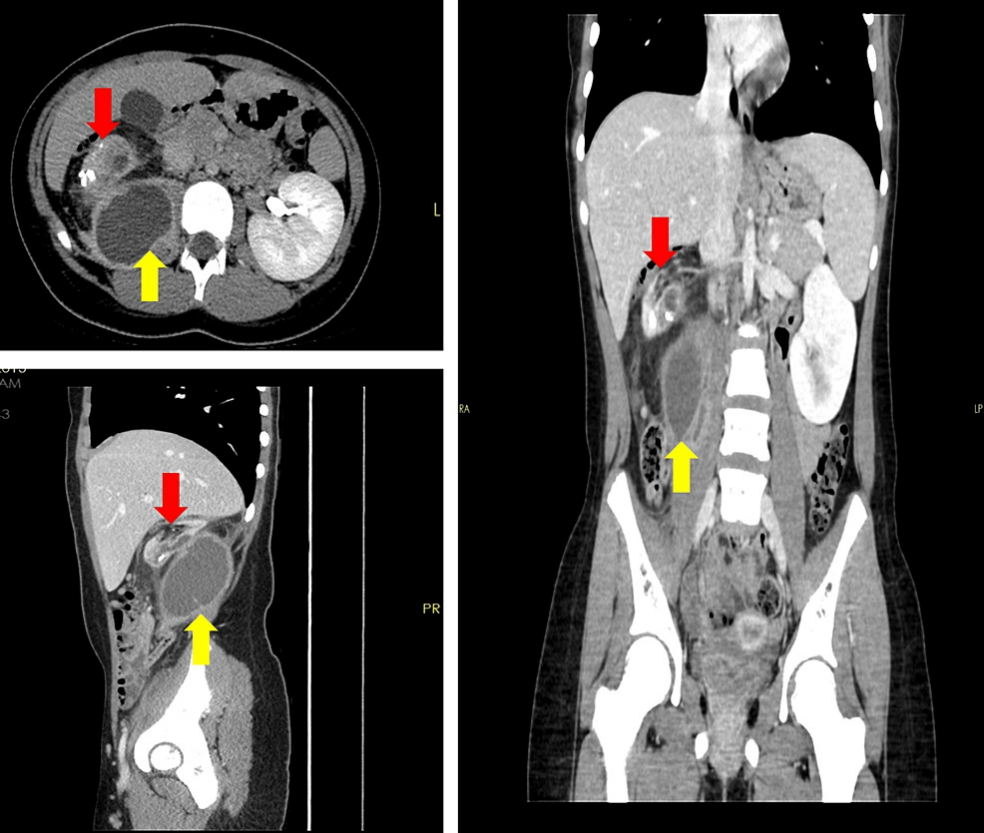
Contrast-enhanced abdominal computed tomography (CT) scan showing global destruction of the right kidney with extensive stones, perinephric/paranephric diffuse inflammatory changes, multiple spots of calcifications, and a large well-defined right psoas abscess. The red arrow represents a destroyed kidney with stones, and the yellow arrow shows pus collection.
